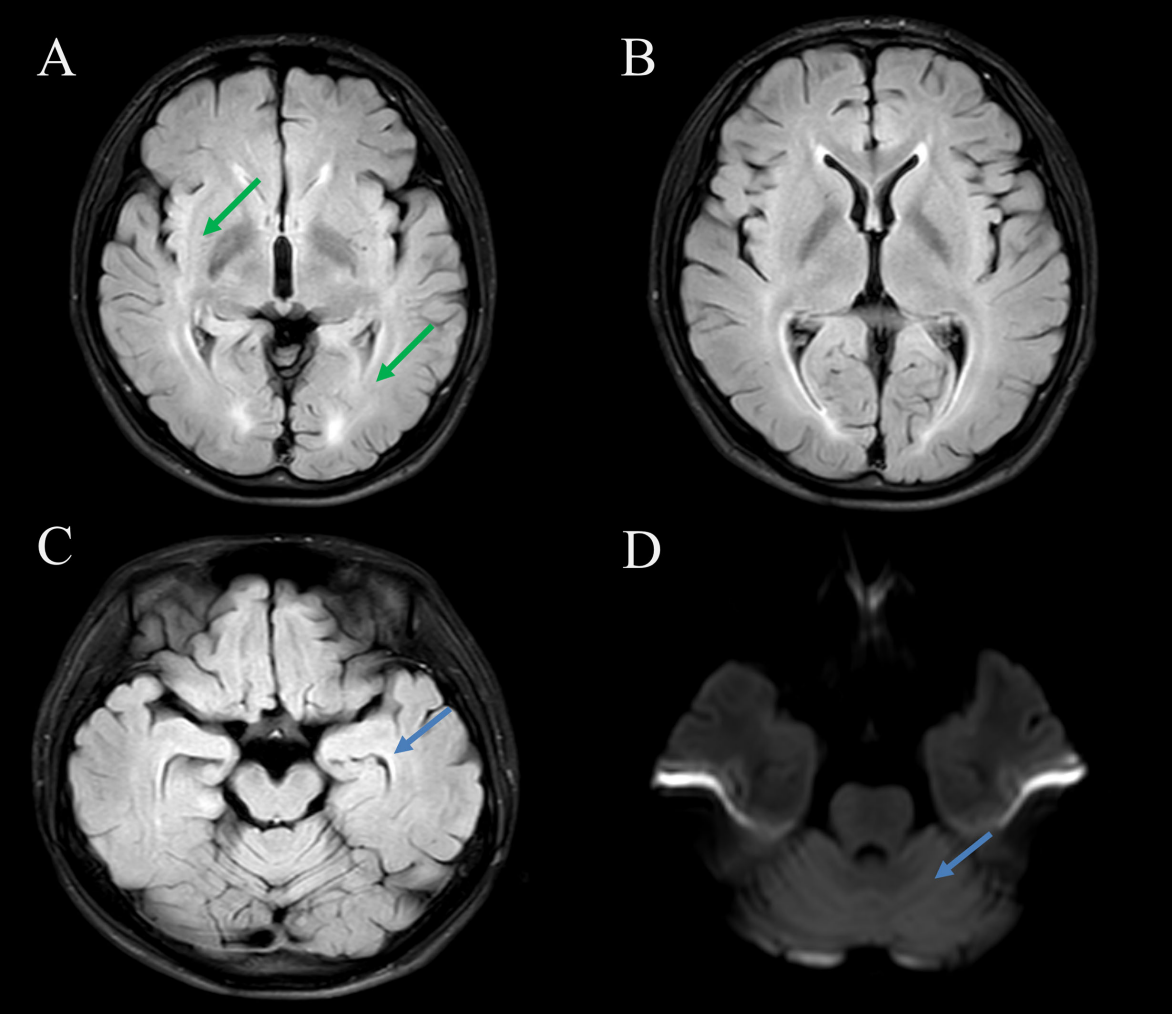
Brain magnetic resonance imaging findings at admission. Magnetic resonance image of the patient’s: (A, B) T2/FLAIR sequences showing subtle hyperintensities in the bilateral insula and periventricular regions (indicated by green arrows). (C) T2-FLAIR sequence reveals no significant involvement of the limbic system, including the hippocampi (indicated by blue arrows). (D) Diffusion-weighted imaging (DWI) shows no apparent abnormalities in the cerebellar regions (indicated by blue arrows).
